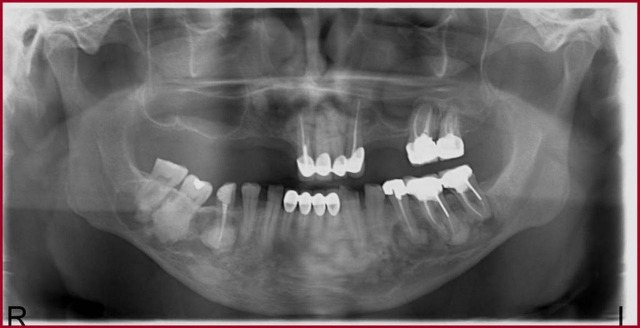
Follow-up panoramic radiograph obtained after an 30-month interval in the patient.
